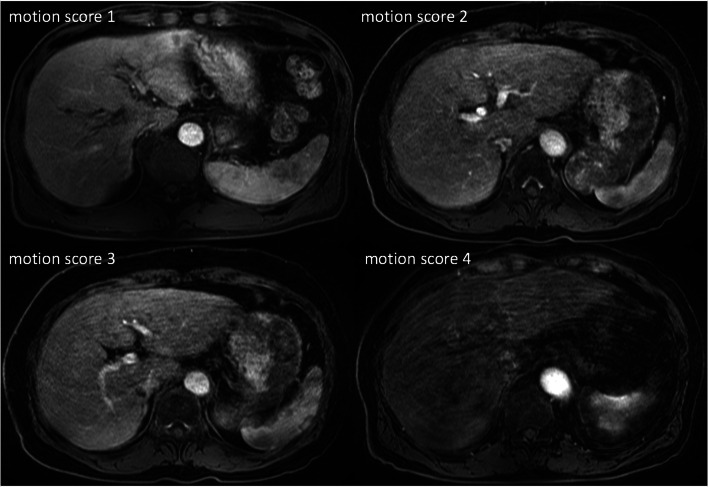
Ranking of motion artifacts in MR imaging on a 4-point scale. Axial T1-weighted transverse MR images following intravenous gadoxetate disodium application. Motion scores were categorized as 1 (no artifact), 2 (mild artifact, no effect on diagnostic quality), 3 (moderate artifact, impeded diagnostic quality), and 4 (severe artifact, non-diagnostic)
